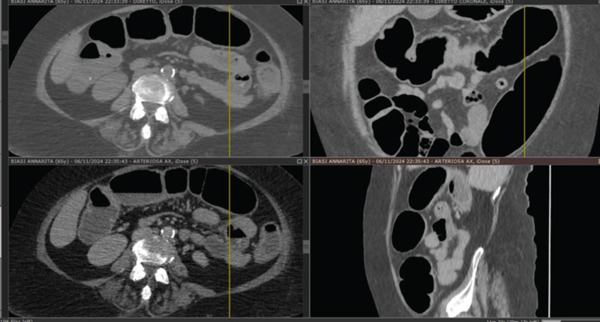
Computed tomography of the abdomen (coronal and axial projections) demonstrating mixed distention of sigmoid colon, transverse colon and rectum with air‐fluid levels. Distended and congested ileal loops with air‐fluid levels are also evident.
